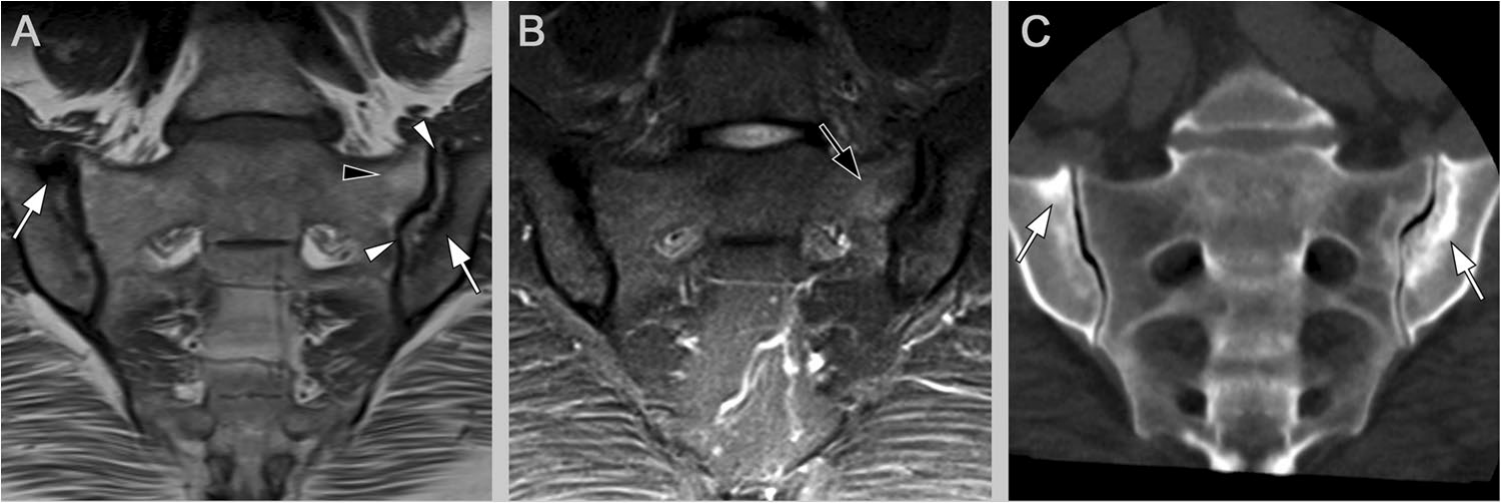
Degenerative joint disease. T1w MRI (A) in a 50-year-old female patient shows sclerosis (white arrows), fat metaplasia (black arrowhead) and fat metaplasia near the articular surface that appears very similar to backfill (fatty metaplasia inside an erosion) which is usually only seen in axSpA. There is also some bone marrow oedema in STIR (B, black arrow). CT (C), however, confirms sclerosis but no other structural lesions, especially no erosions. Therefore, and in accordance with clinical findings, the diagnosis of axSpA was refuted, and osteoarthritis/degenerative joint disease was confirmed
